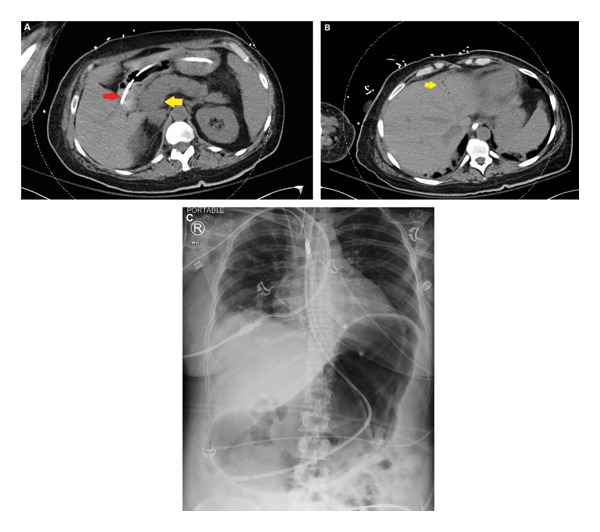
(A) CT scan of the abdomen with yellow arrowhead showing pancreatic head with edema and evidence of acute pancreatitis and red arrowhead showing the NG tube tip in the duodenum. (B) CT scan of the abdomen with yellow arrowhead showing pneumobilia. (C) Abdominal X‐ray showing distended stomach after NG tube insertion.
